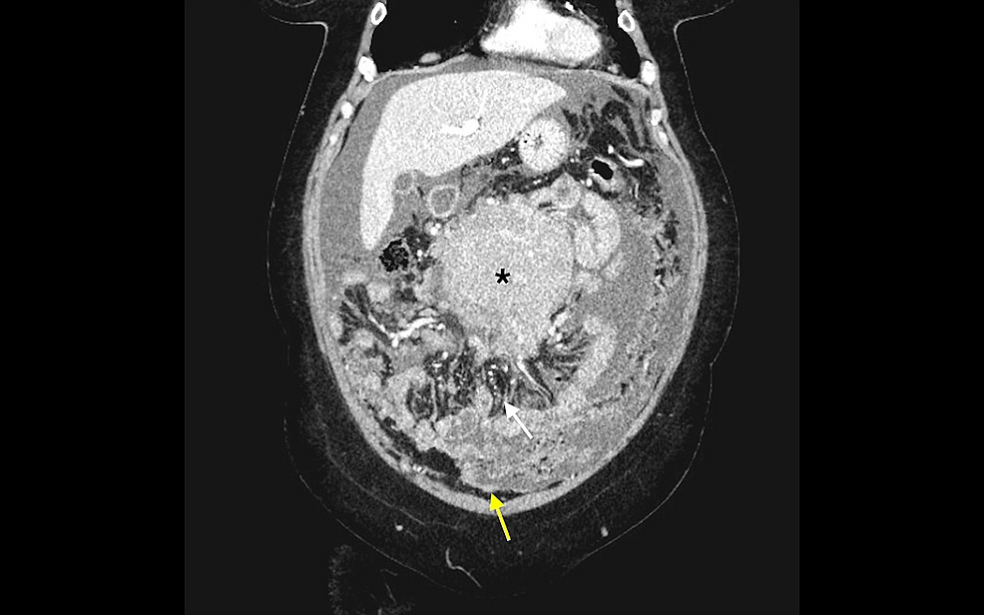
Computed tomography of the abdomen (coronal section) CT abdomen describes a large mid-abdominal mass (black asterisk), mesenteric thickening (yellow arrow), and peritoneal thickening with stellate appearance (white arrow) suggesting peritoneal carcinomatosis.
